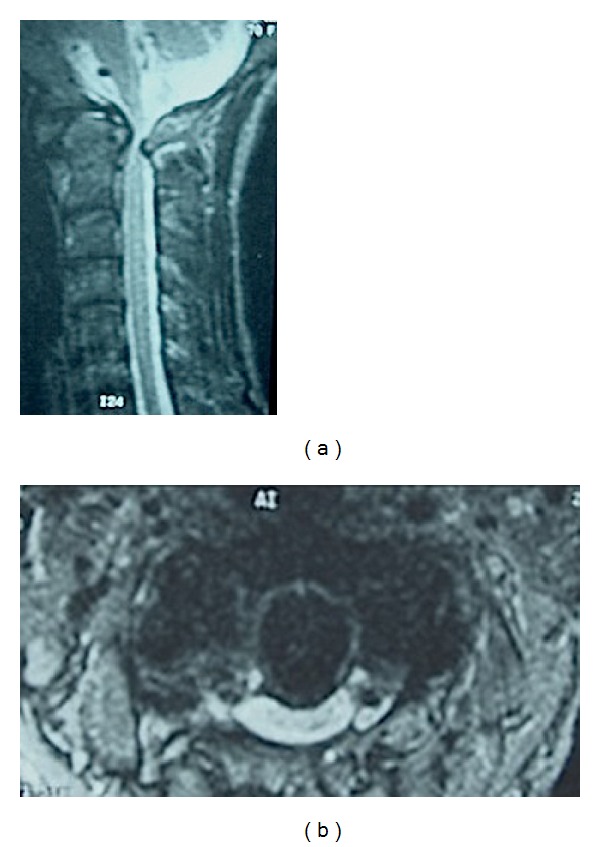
Sagittal (a) and axial (b) T2-weighted image MRI scans of the cervical spine demonstrating hypoplastic posterior arch of the atlas causing severe canal stenosis and spinal cord compression.
